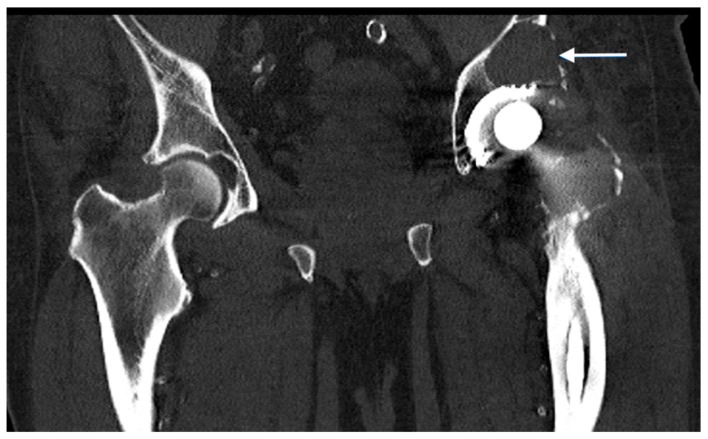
Computed tomography of a patient with an aseptic loosening of a hip endoprosthesis and an acetabular abrasion granuloma (indicated by the white arrow).
